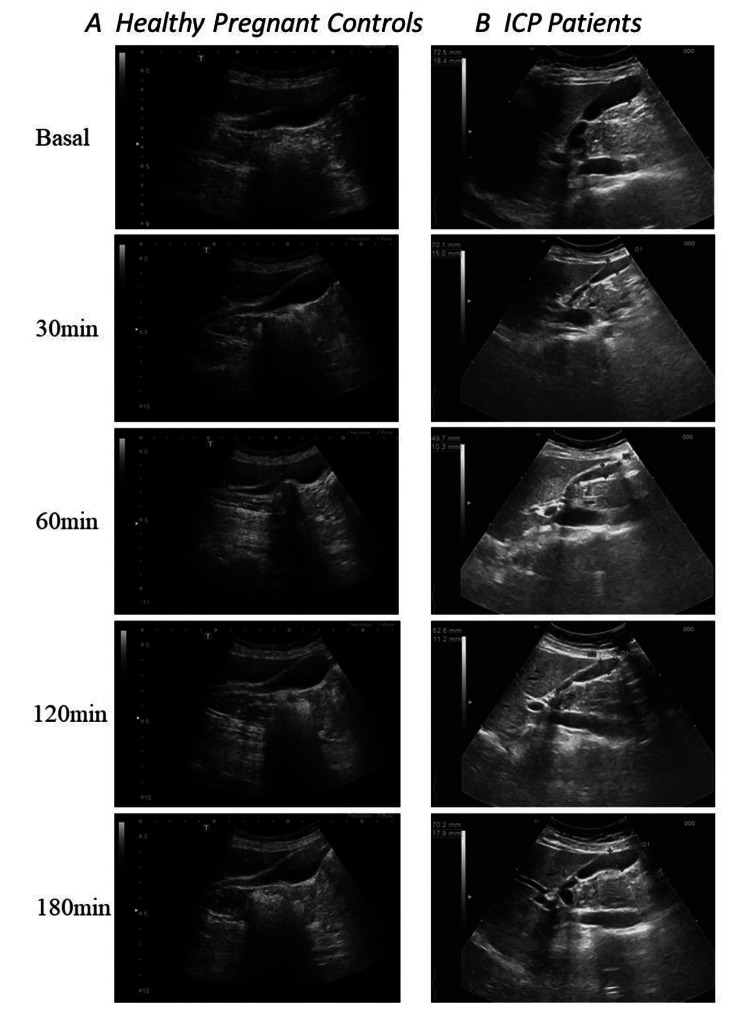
Ultrasonographic measurement of the gallbladder volume. A, Ultrasound examination of the gallbladder volume in healthy pregnant women after overnight fasting and 30, 60, 120, and 180 min after a liquid test meal of 200 mL; B, Ultrasound examination of the gallbladder volume in ICP patients
